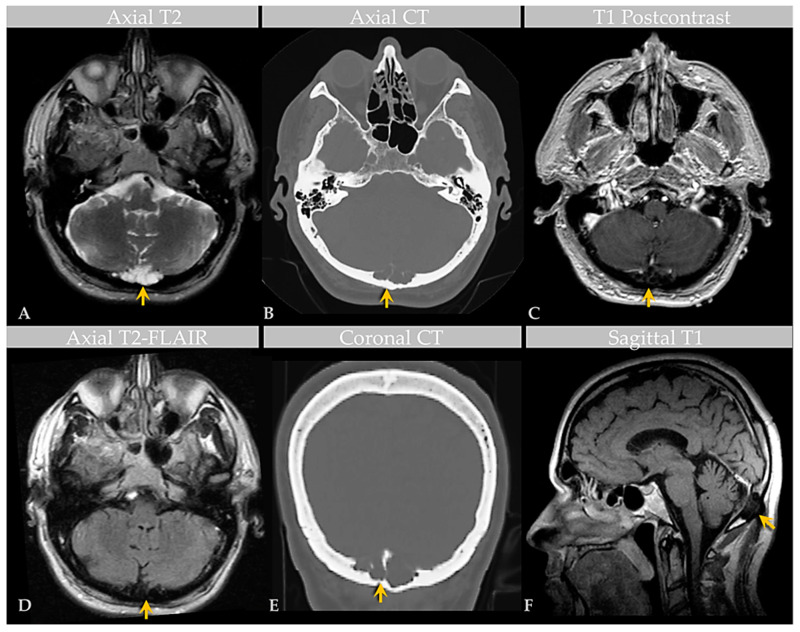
Case 2: Imaging of an individual with GAGs involving the confluence of sinuses. MRI (A) and CT (B) images show a GAG measuring over 3.6 cm centered in the region of the torcula horophili eroding through the diploic space and remodeling the outer cortex of the occipital bone (arrows). Note that the diploic space is expanded. There are thin, nonenhancing septae within the AG, as shown on postcontrast T1-weighted (C) and T2-FLAIR (D) MRI images, causing internal signal heterogeneity. Features are also demonstrated in the coronal CT image (E) and sagittal T1-weighted image (F). Images are from the same individual depicted in Figure 3.
